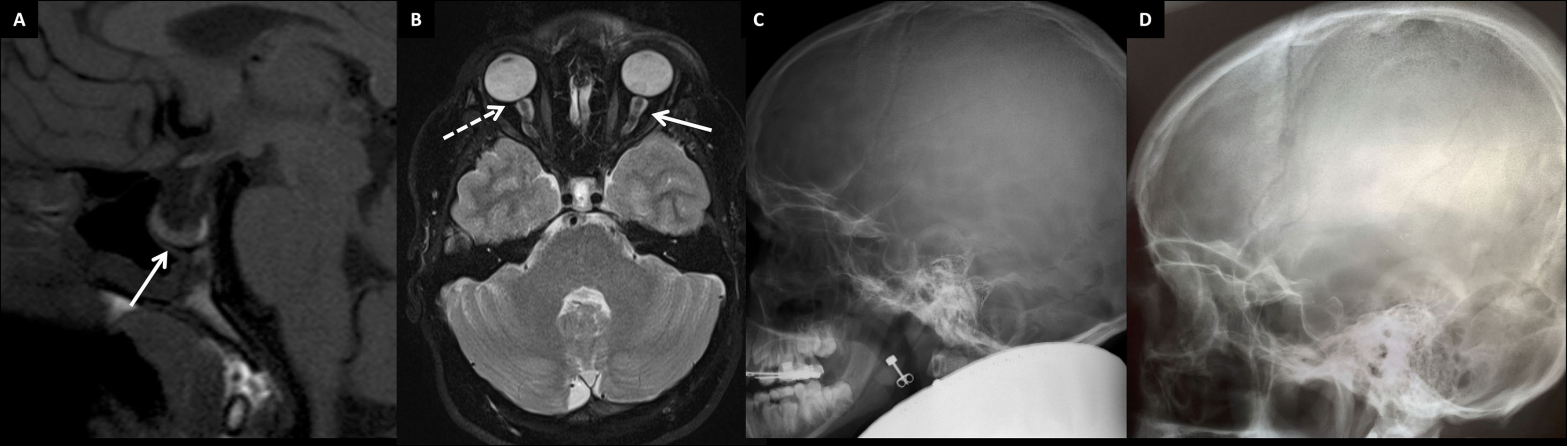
Brain structure findings in two patients with the novel skeletal dysplasia. (A) Non-enhanced T1 sagittal MR image showing an enlarged pituitary fossa filled mainly with CSF and pituitary tissue lining the sellar floor (arrow). The bright spot behind the adenohypophysis represents neurohypophysis at the normal position in the 13-year-old female index (III-1). (B) Tortuous optic nerves with distended perioptic nerve sheaths (arrow), and optic globe flattening (flattening of the posterior part; dashed arrow) in the 13-year-old female index. Skull radiographs of the female index (III-1) (C) and her father (II-1) (D) showing an enlarged pituitary fossa in both.
